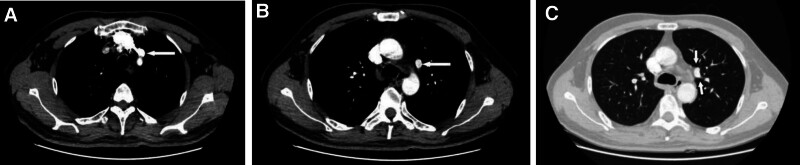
CT confirmed a partial anomalous pulmonary vein connection of the left upper lobe. (A, B) In the mediastinum windows, ectopic drainage of the upper pulmonary veins can be observed in the general direction: blood from the S1 + 2 + 3 vein in the left upper lobe of the lung returns to the left cephalobrachial vein along the superior border of the aortic arch. (C) Variant pulmonary vein bifurcation is seen in lung window. CT = computed tomography.
